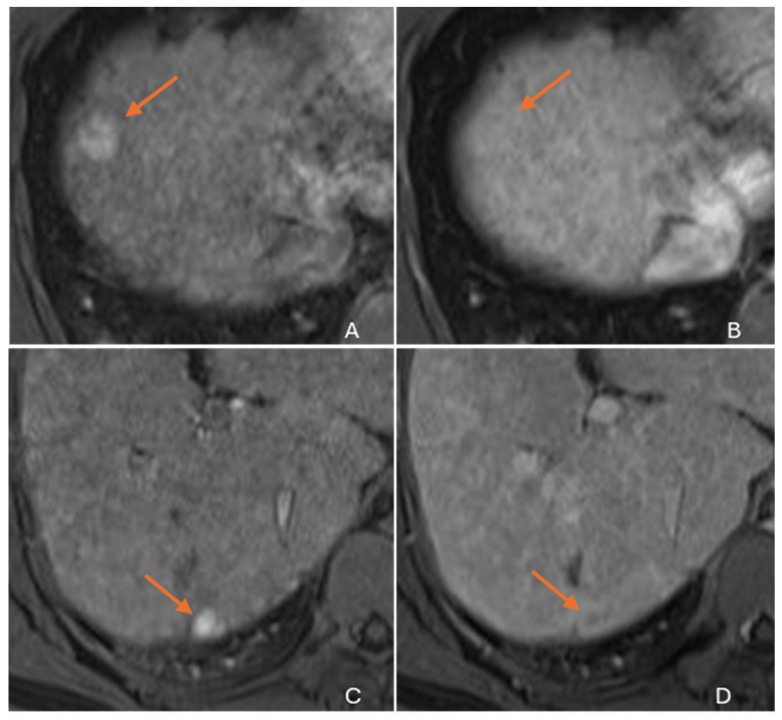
Axial gadolinium-enhanced MRI images in a 43-year-old male with HCV cirrhosis and pathologically proven cHCC-CCs on explant. A 2 cm cHCC-CC (arrows) at the anterior subcapsular border of segment 8 shows LR 4 features with diffuse arterial hyperenhancement (A) and is isosignal on the delayed phase (B). At a lower slice, a 1.2 cm cHCC-CC (arrows) at the posterior subcapsular border of segment 7/6 shows LR 5 features with diffuse arterial hyperenhancement (C) followed by delayed phase washout (D).
